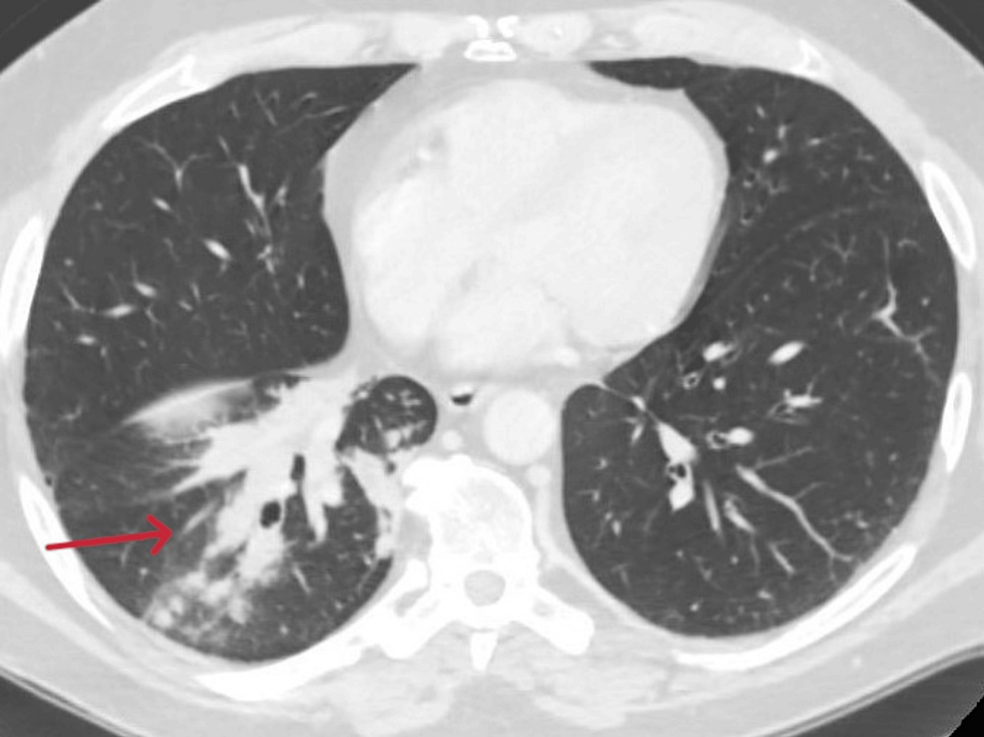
CT scan of the patient at diagnosis of EGPA: bronchiectasis associated with ground-glass pulmonary infiltrates CT: computed tomography, EGPA: eosinophilic granulomatosis with polyangiitis
